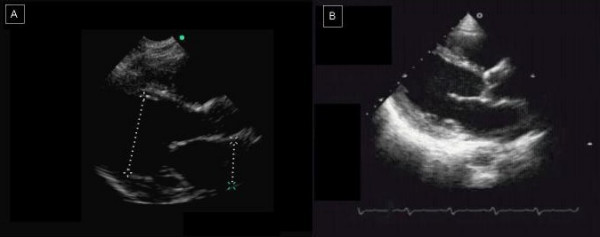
A case of dilatative cardiomyopathy. On the left (A), HHD image in a parasternal long-axis view obtained with HHD. On the right (B), the same patient evaluated with SED.
